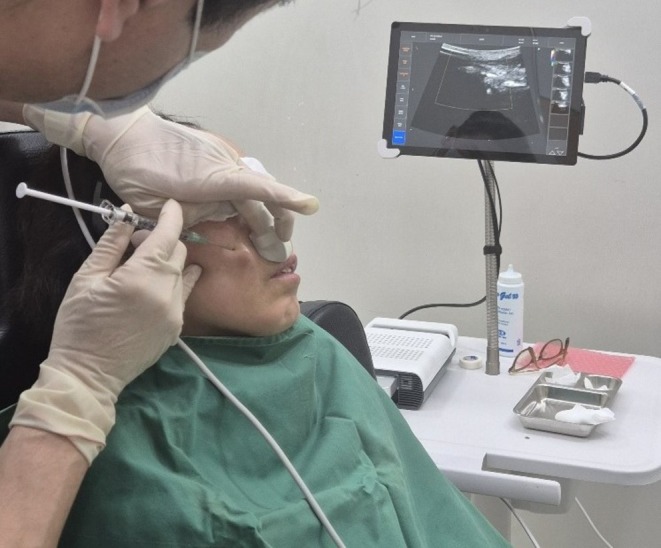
Ultrasound‐guided hyaluronic acid filler injection. Detection of the cannula in the monitor.
